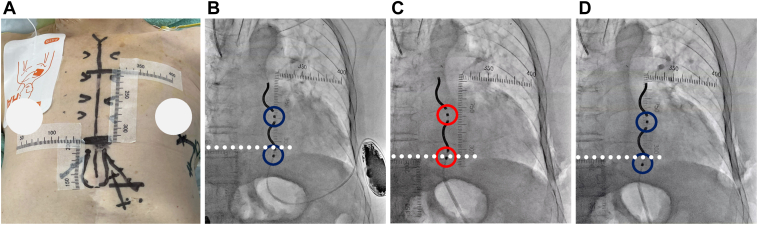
Intraoperative fluoroscopy. A: The top of the cardiac silhouette, xiphisternal junction, and both parasternal borders were visualized using radiopaque tape on the skin surface. Anteroposterior fluoroscopic views show (B) the initial substernal lead position with the ring 1 and ring 2 electrodes highlighted (*blue circles*), (C) the substernal lead repositioned slightly rightward (*red circles*), and (D) the final position, where the R-wave amplitude remained below the success criteria despite securing the ring electrodes being near the initial position (*blue circles*). Ring 2 was located inferior to the level of the xiphisternal junction (*white dashed line*).
